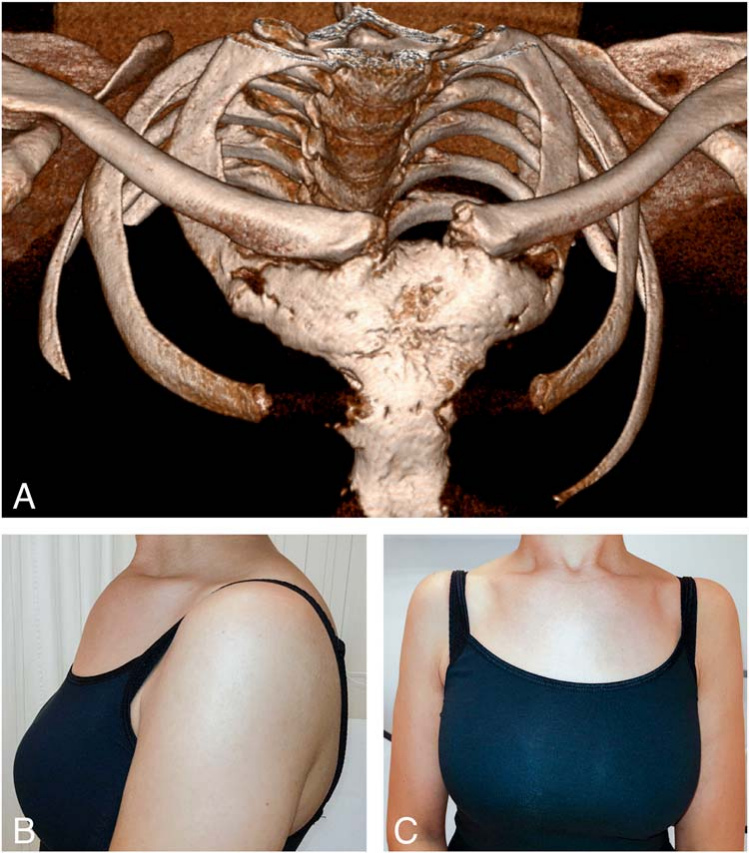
(A) 3D image reconstruction of a CT scan of the sterno-costo-clavicular region in a different patient with CNO showing bilateral hyperostosis of the manubrium sterni at the level of the first rib forming a large bony costo-manubrial bridge. Hyperostosis of the proximal right clavicula and ankylosis of the manubrio­ sternal joint can also be seen. (B and C) Frontal and lateral clinical images obtained at diagnosis in the same patient showing bone enlargement in the SCC region of the anterior chest wall.
